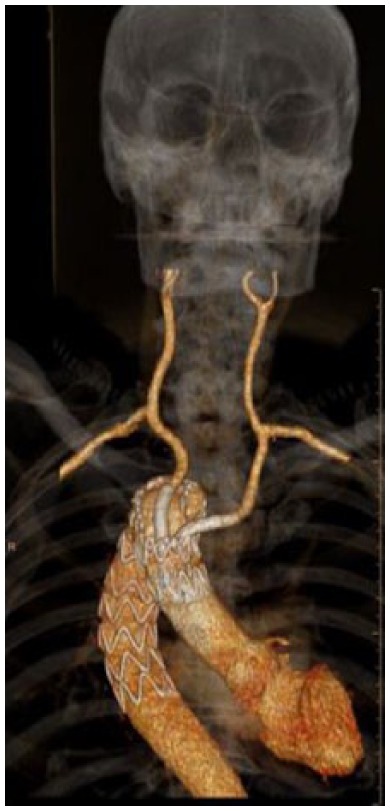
Three-dimensional volume rendering CT angiogram at 3 months.
